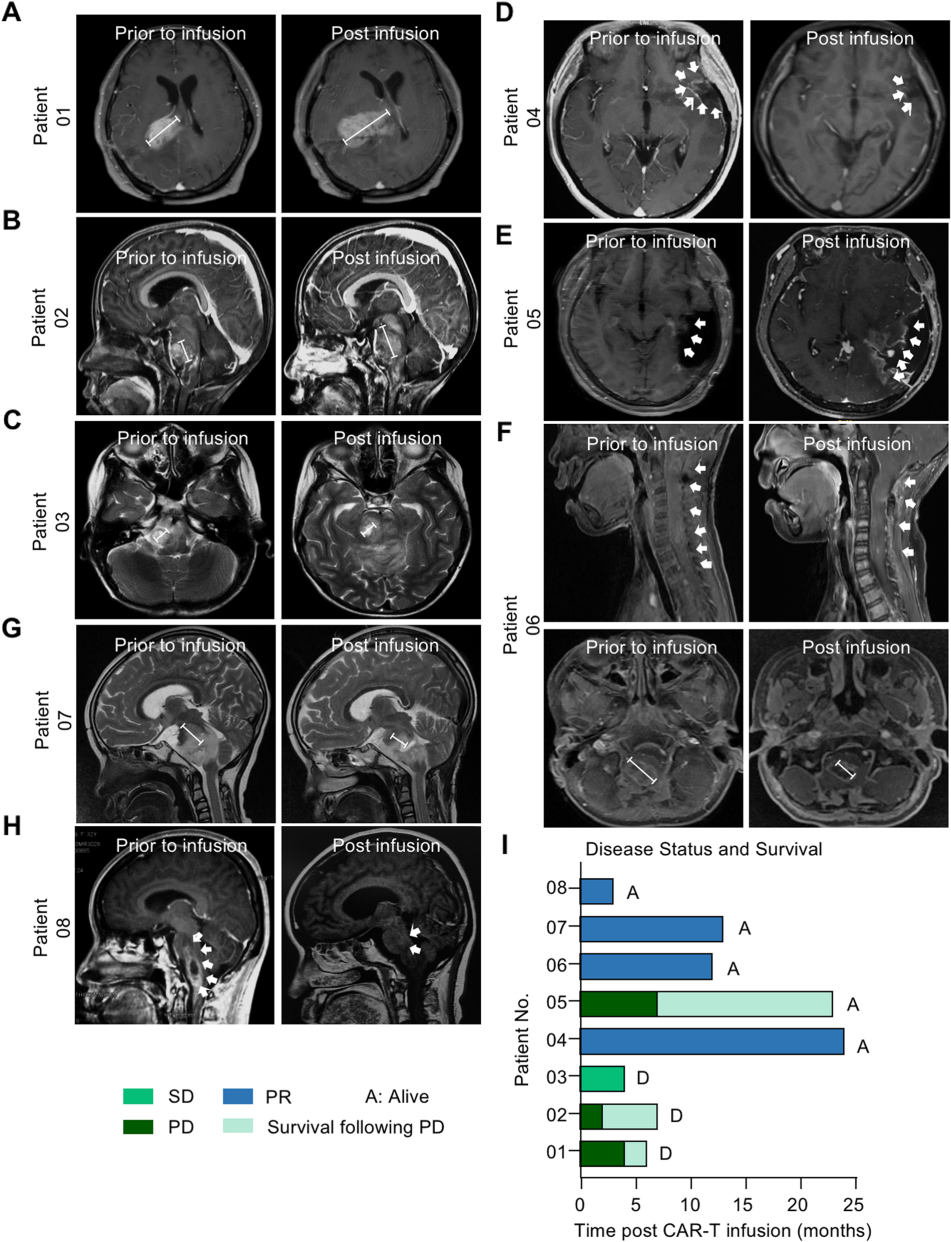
MRI scan of brain and overall survival post GD2 specific 4SCAR-T cell infusion. A-H Magnetic resonance imaging (MRI) scan of the brain before and 4 weeks after GD2 specific 4SCAR-T cell infusion. I Swimmer’s plot describing disease status and overall survival for each patient. PD, progressive disease. SD, stable disease. PR, partial response. A, Alive. D, Dead
